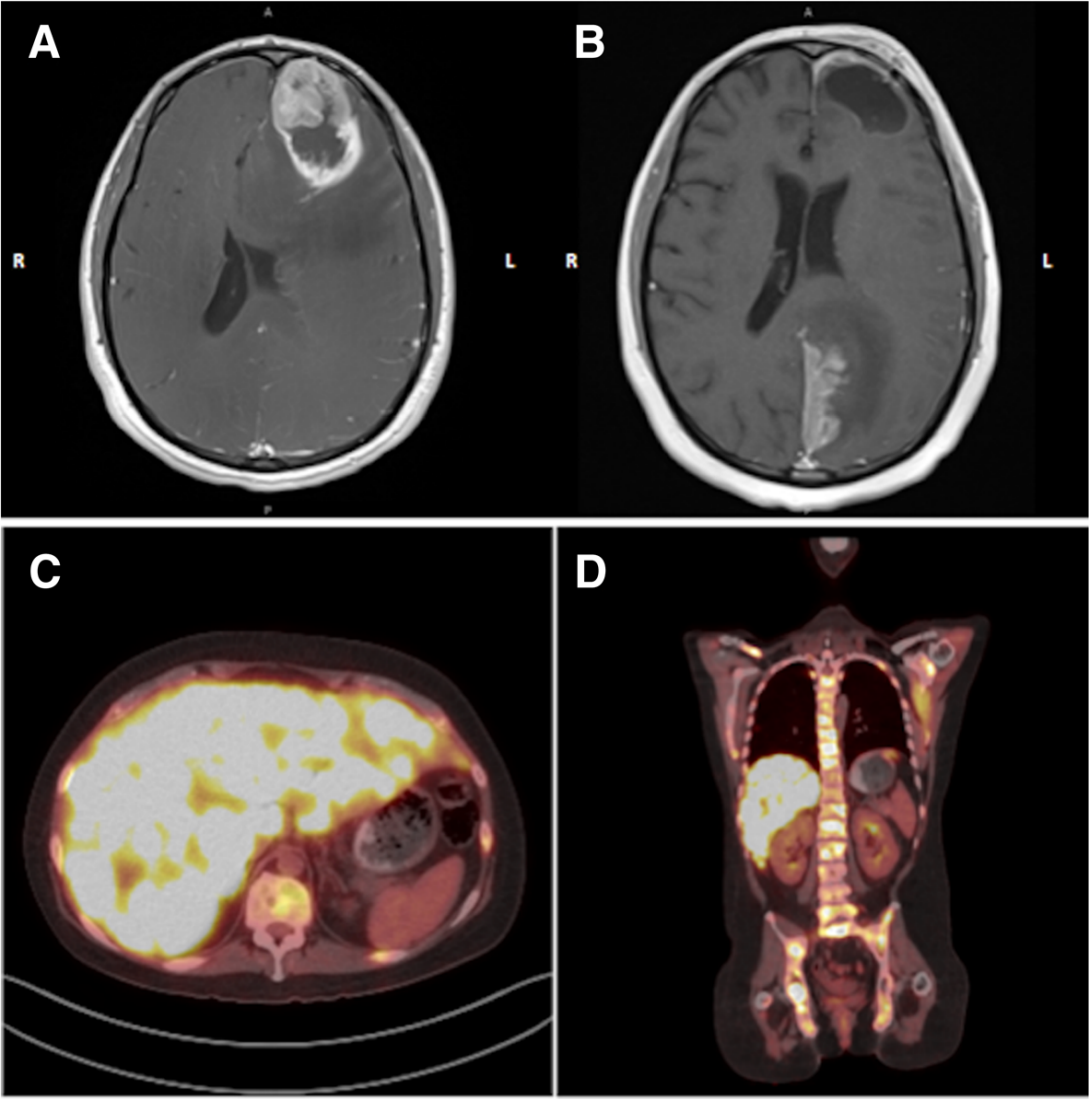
Axial MRI brain post administration of gadolinium a on initial presentation with a large contrast enhancing lesion and a necrotic core in the left frontal lobe with mass effect. b Images 2 weeks after completion of radiation therapy showing an enhancing lesion involving the paramedian left parietal-occipital lobe extending to the superior surface of the left cerebellar tentorium. c axial and d coronal FDG PET scan with hypermetabolic lytic bony lesions throughout the axial and proximal appendicular skeleton as well as innumerable hyperdensities in the liver concerning for metastatic disease
